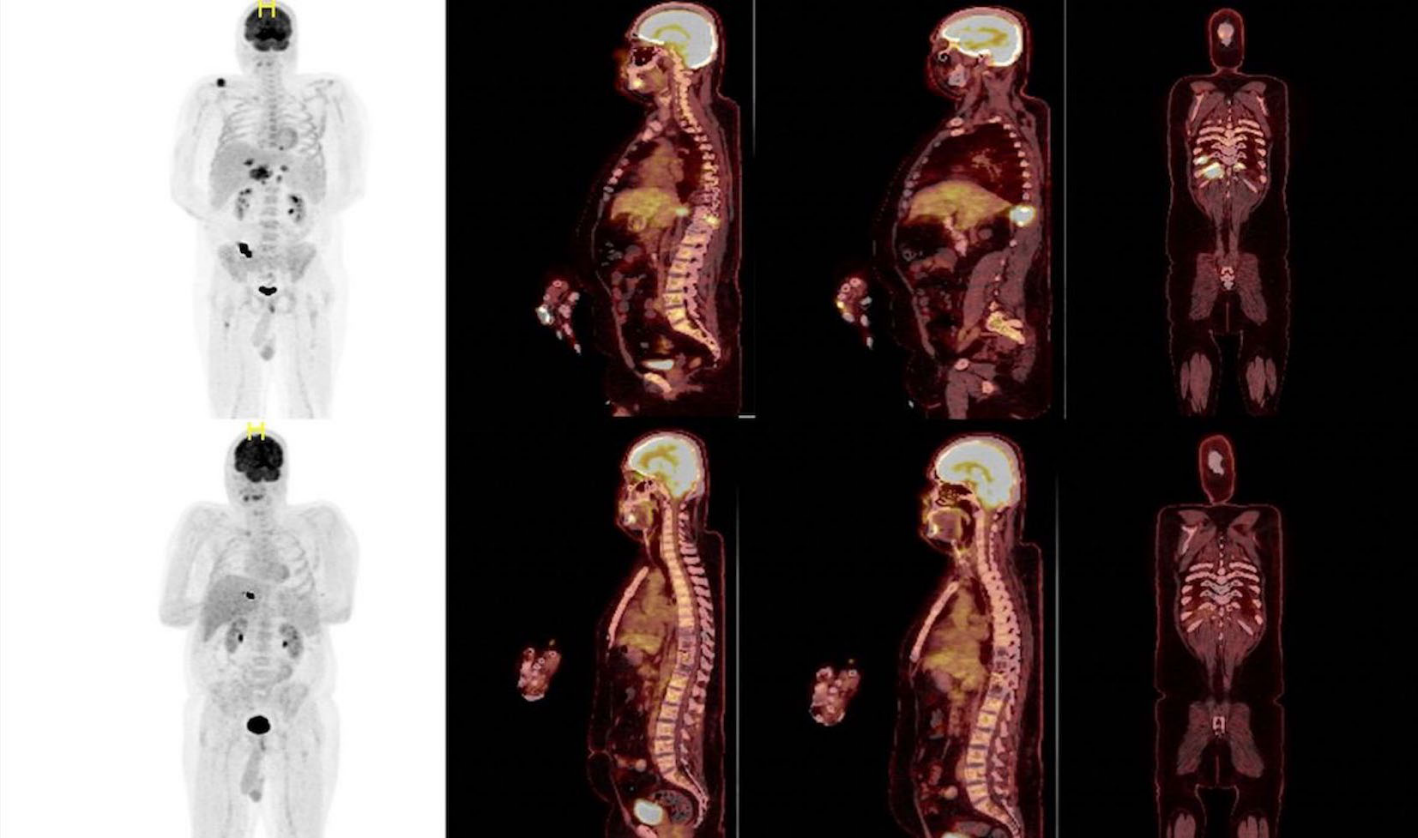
PET CT images are showing a complete remission before (on the top) and after (bottom) Elranatamab therapy.
